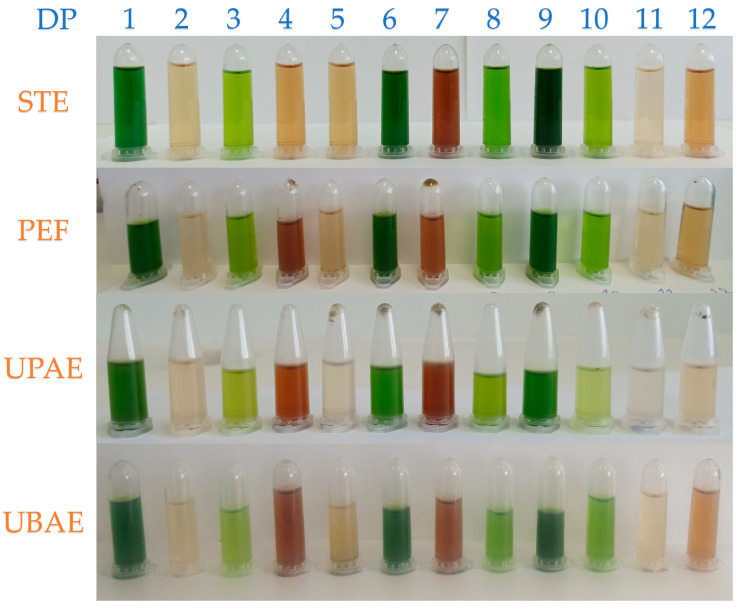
Sample extracts were obtained using four (4) different extraction techniques and twelve (12) design points (DPs) from the Plackett–Burman design. STE—stirring extraction; PEF—pulsed electric field-assisted extraction; UPAE—ultrasonic probe-assisted extraction; UBAE—ultrasonic bath-assisted extraction.
